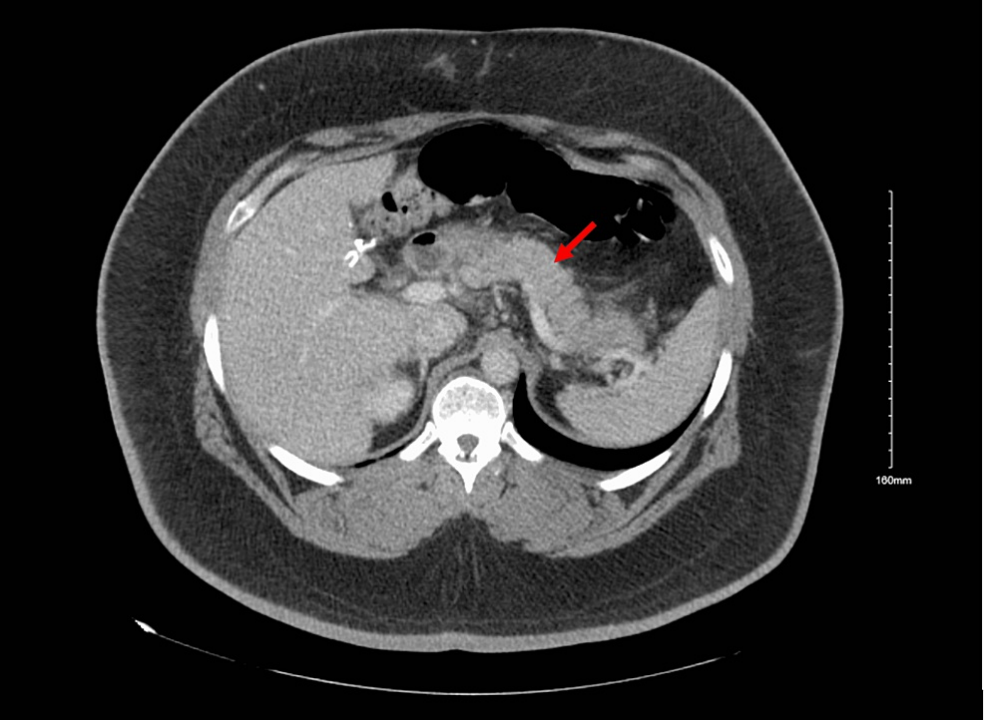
CT abdomen Computerized tomography of the abdomen showed peripancreatic stranding and haziness (arrow) suggestive of acute pancreatitis.
